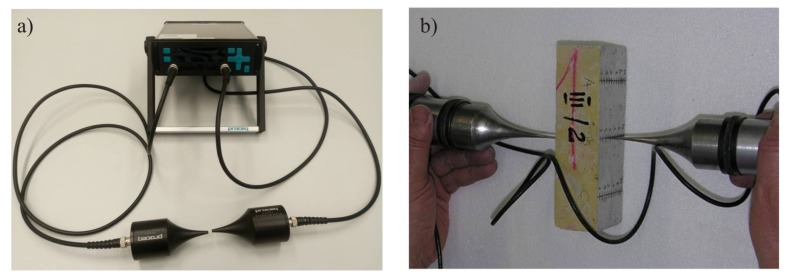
Example view: (a) head and probe for ultrasonic testing; (b) floor sample tested using the ultrasonic method.
